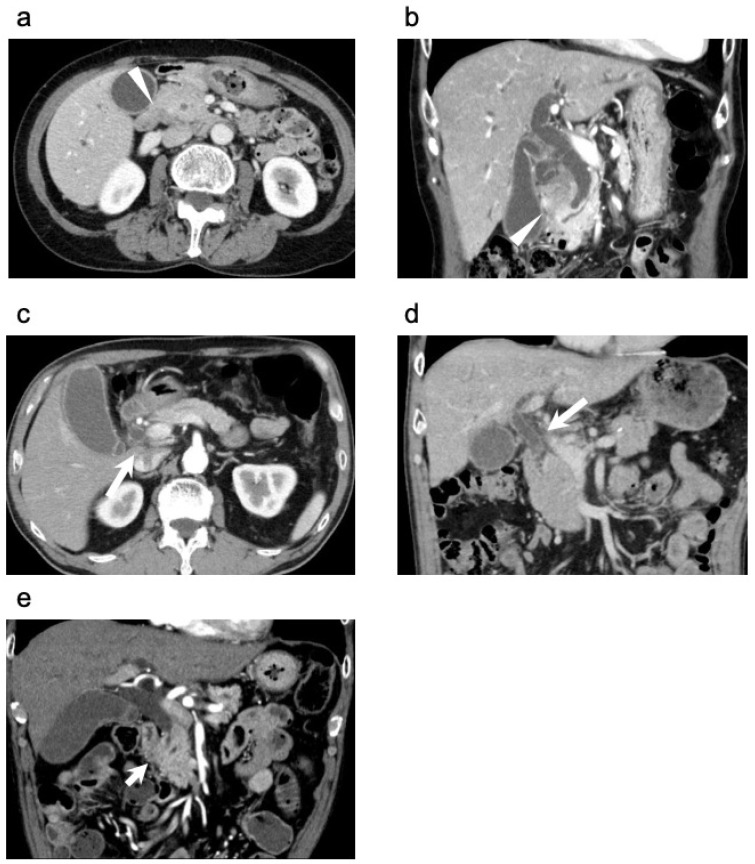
Preoperative contrast-enhanced computed tomography findings in a representative patient initially diagnosed with distal cholangiocarcinoma: (a) Axial view showing a lesion extending beyond the bile duct (arrowhead). (b) Coronal view also showing extension of the lesion beyond the bile duct (arrowhead). (c) Axial view revealing soft tissue density along the hepatoduodenal ligament (large arrow). (d) Coronal view confirming soft tissue density extending longitudinally along the hepatoduodenal ligament (large arrow). (e) Axial view showing obstruction of the main pancreatic duct (small arrow).
